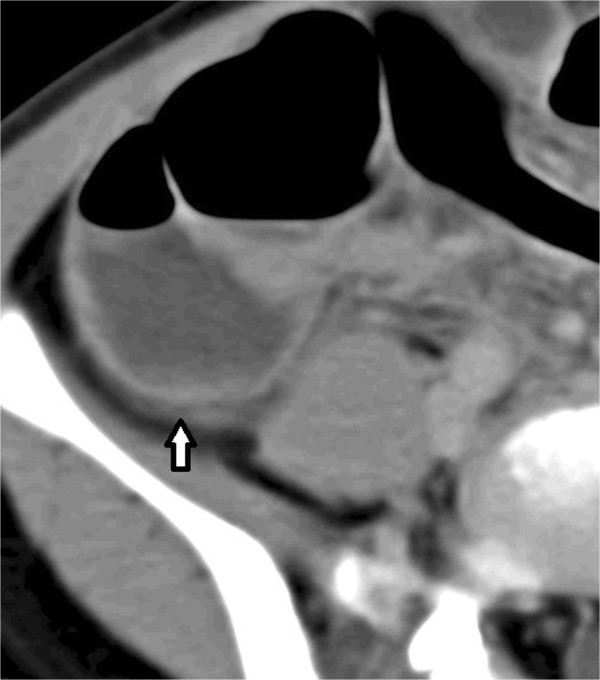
Transverse CT image of 6-year-old patient with complicated appendicitis and ileus. Identification of RPS and other extraperitoneal spaces was difficult due to the patient’s younger age and ileus. Combined fascial plane (white arrow) involvement was observed.
